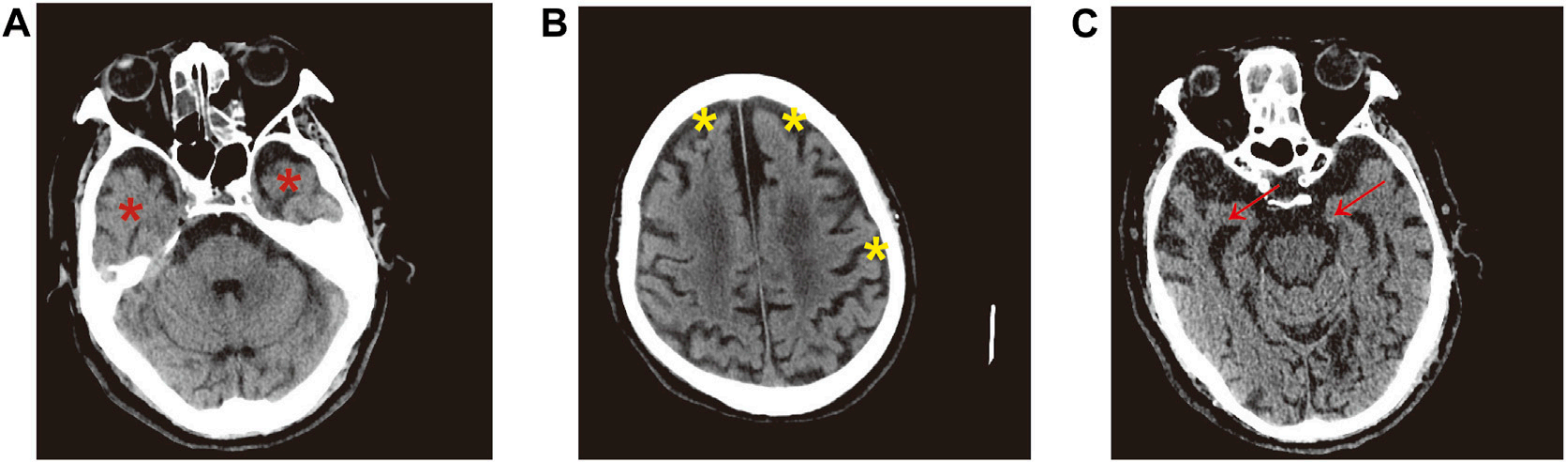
Representative brain CT images of AD patients. (A) Red asterisk indicates obvious atrophy of temporal lobe and dilated temporal horn in the bilateral brain. (B) Significantly atrophied cerebral gyrus, yellow asterisk denotes deepened sulcus. (C) Red arrow represents distinctly reduced transverse diameter of hippocampus in the bilateral brain.
